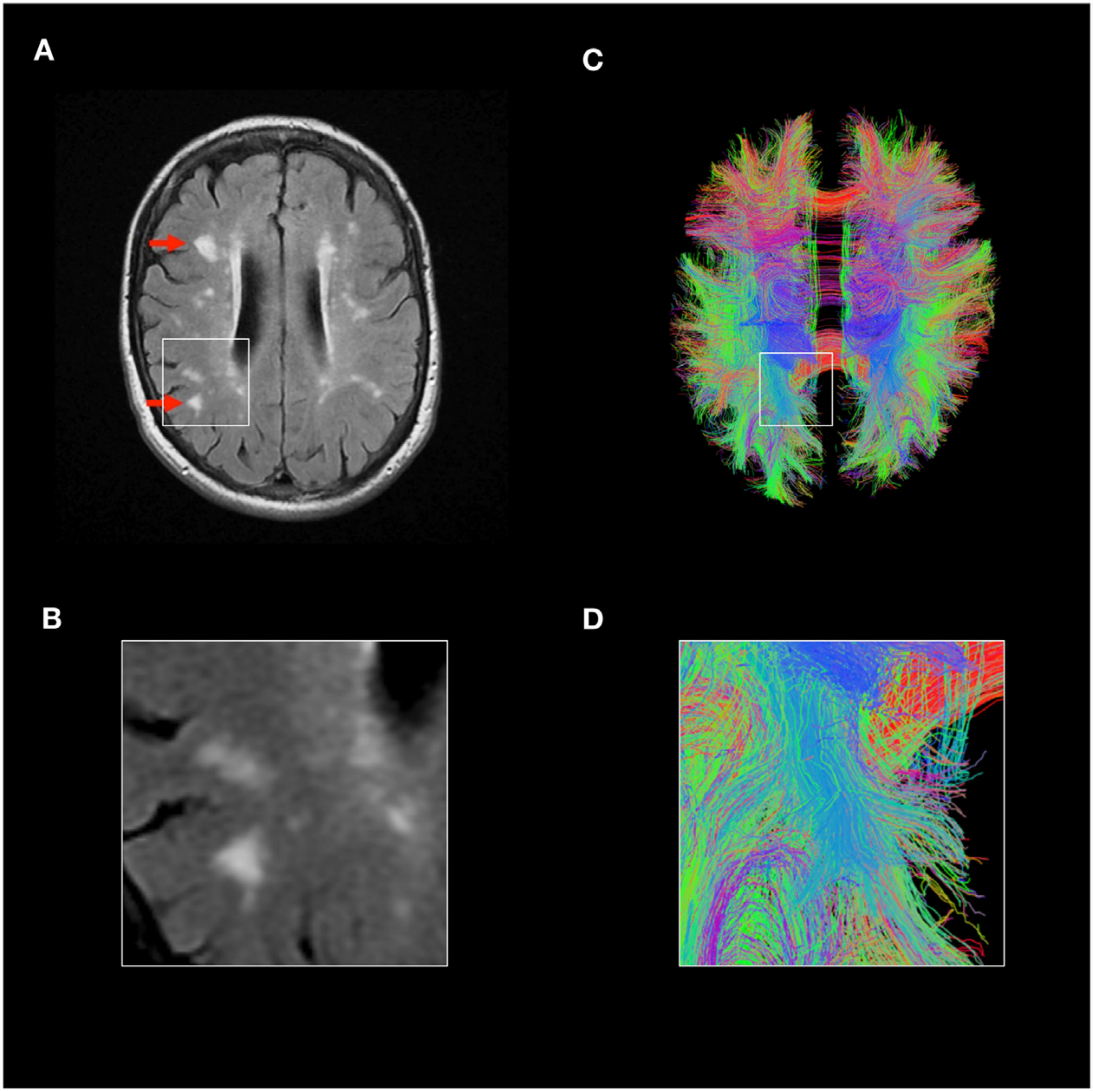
Magnetic resonance imaging (MRI) imaging in lupus brain disease. (A,B) Fluid-attenuated inversion recovery MRI scan of a representative individual with lupus, showing accelerated cerebral small vessel disease, highlighted red arrows. (C,D) Advanced MRI techniques such as diffusion tensor imaging and tractography can allow identification of individual white matter tracts and parameters such as mean diffusivity can identify microstructural disease. Tractography images of lupus patient shown, each line represents individual white matter tract. Credit: Mark Bastin, Joanna Wardlaw, and Stewart Wiseman.
